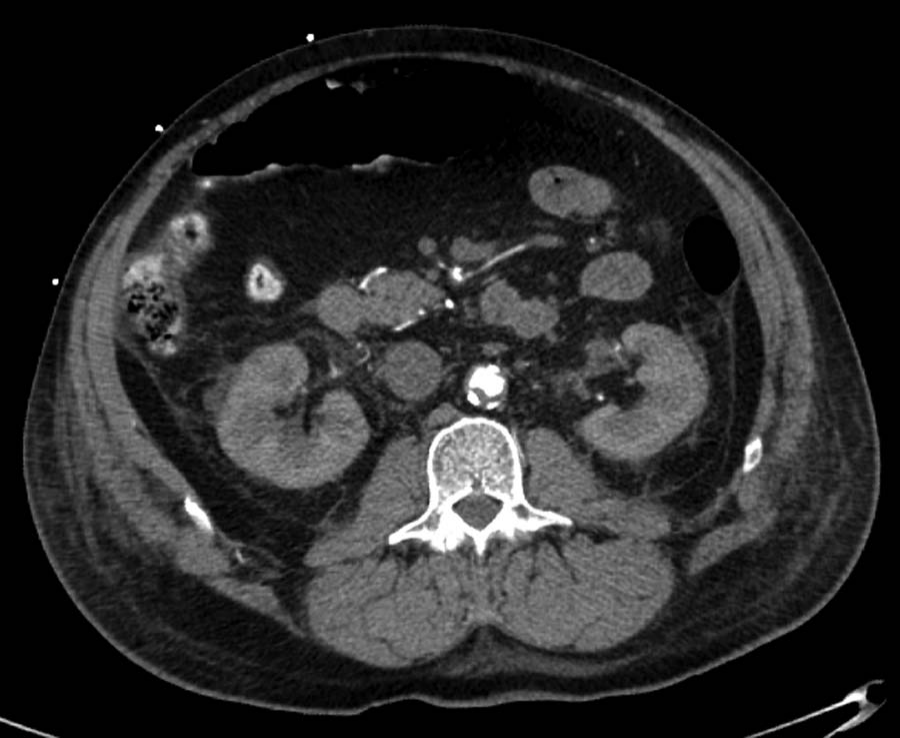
Computed tomography angiogram of aorta demonstrating intraluminal thrombus of abdominal aorta.
